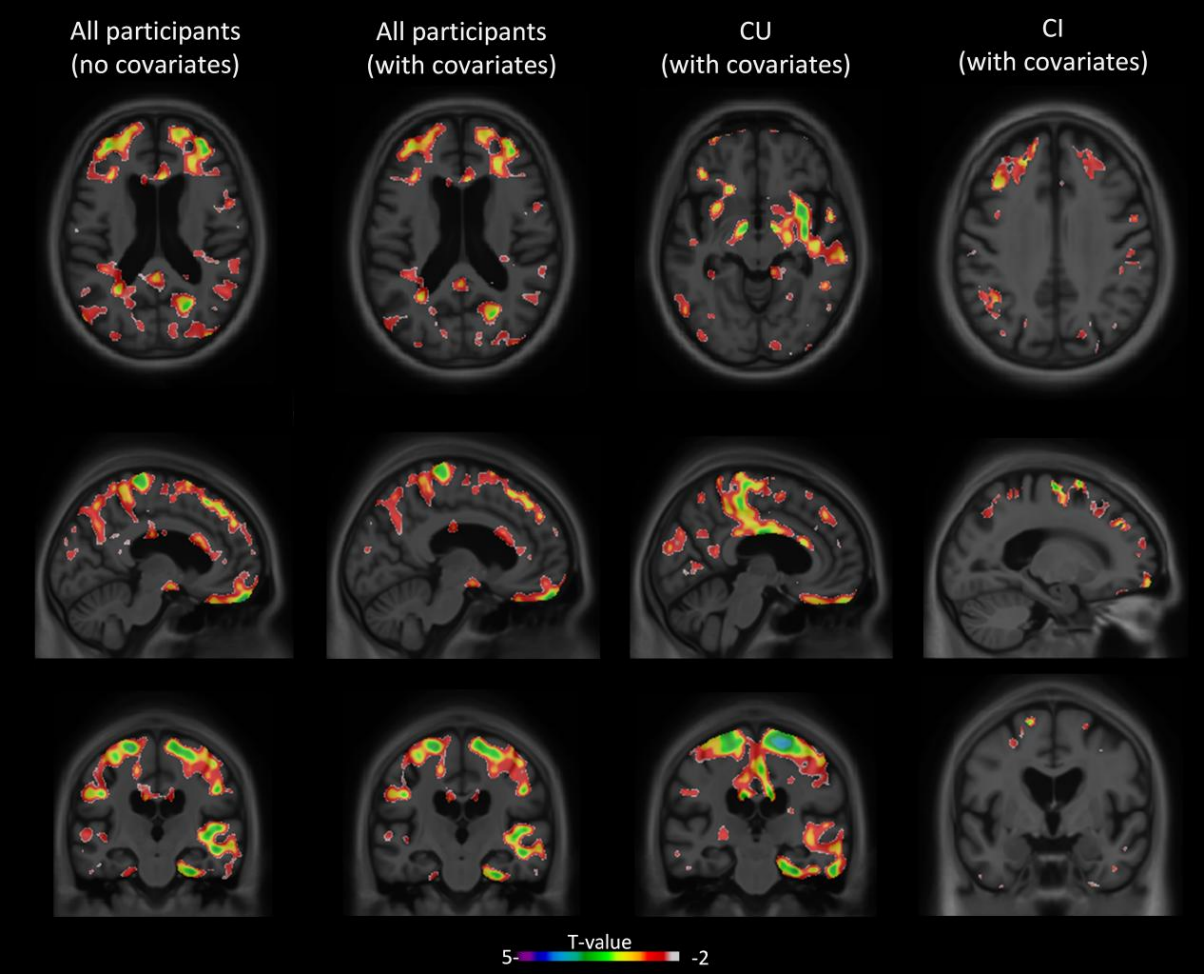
Carriers of the *FNDC5* rs1746661(T) SNP present brain glucose hypometabolism. Figure shows transversal (upper row), sagittal (middle row) and coronal (lower row) images showing FDG-PET metabolism in *FNDC5* rs1746661(T) carriers compared to non-carriers in all participants without adjustments for covariates (left) and with adjustments for covariates (age and sex) (centre left). Similarly, in CU participants’ *FNDC5* rs1746661(T) carriers had reduced FDG-PET metabolism as compared to non-carriers (adjusting for covariates; centre right). CI participants’ *FNDC5* rs1746661(T) carriers also had reduced FDG-PET metabolism as compared to non-carriers (adjusting for covariates; right) but to a much lesser extent than that observed in CU participants. *t*-values are shown as a colour scale in each image, and significant results have a *t*-value smaller than or equal to −3.1.
